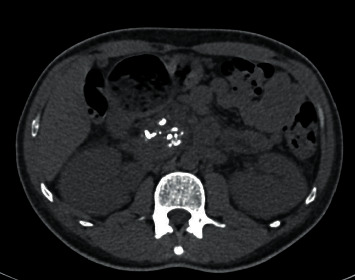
Axial noncontrast CT through the head of the pancreas shows coarse calcifications within the pancreatic parenchyma more pronounced in the head and uncinate process and are also seen in bilateral perinephric fat stranding.
